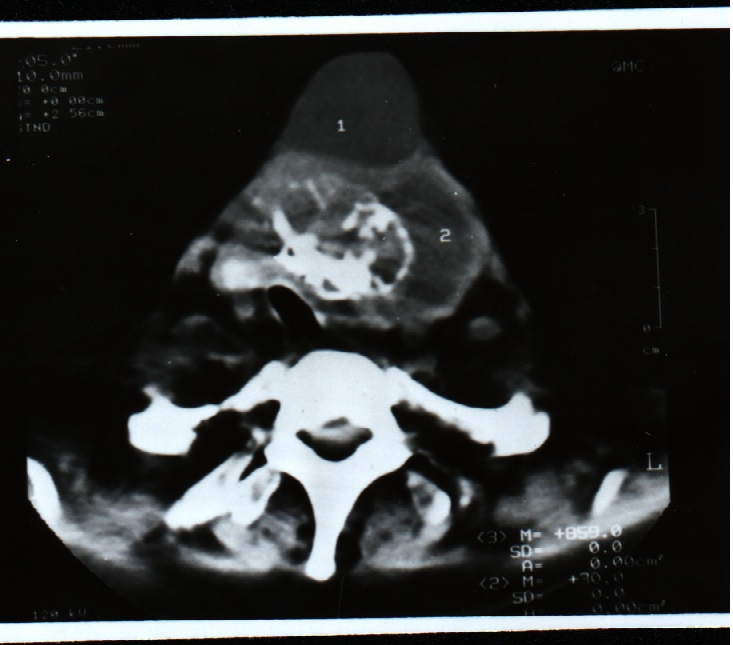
Case 1: Computed tomography showing the growth with pressure on the trachea.
